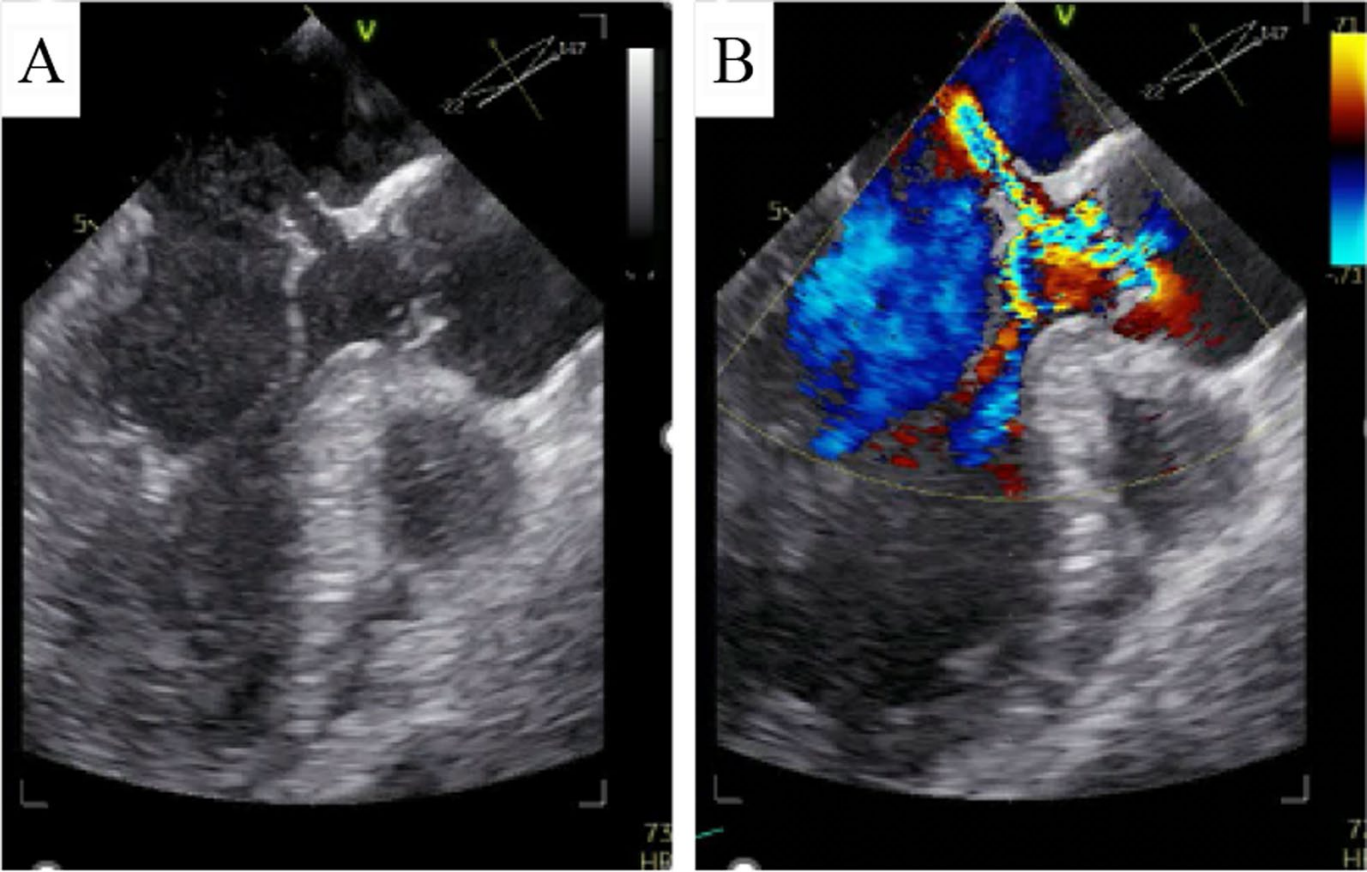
A Transesophageal echocardiography (TEE) showed the pseudoaneurysm with a perforation at the AML. B TEE revealed moderate AR due to right coronary cusp prolapse and severe regurgitant flow from left ventricular outflow tract to the left atrium in systole
